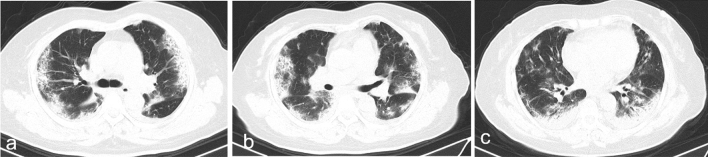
Transverse CT scans in a man infected with SARS-CoV-2. Scan obtained on 10th day from onset of symptoms shows diffuse, heterogeneous consolidation that affected bilateral, subpleural lung parenchyma. The patient was discharged from hospital 7 days the scan.
